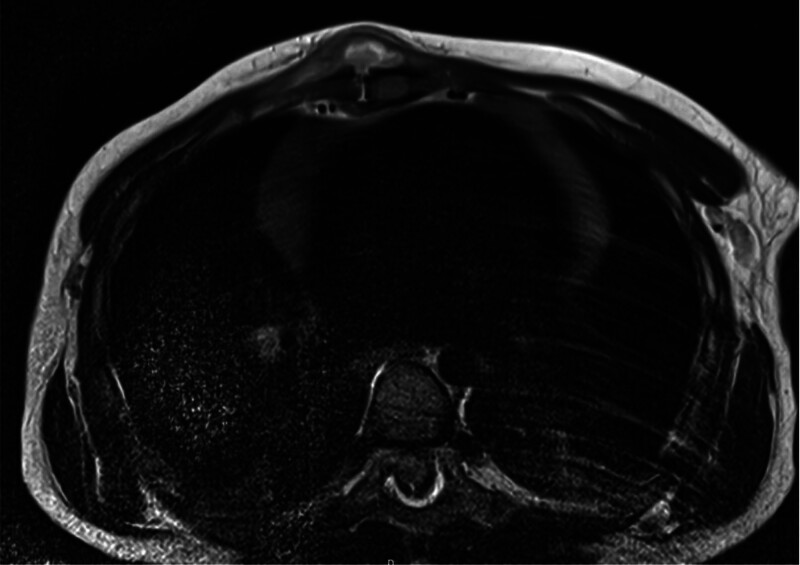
Axial MRI showing presternal abscess formation reaching through to the retrosternal space.
